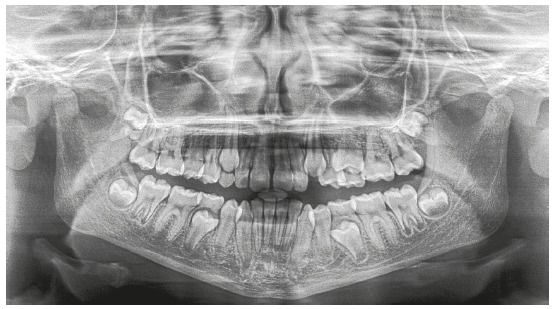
Case 1: Baseline panoramic radiograph.
